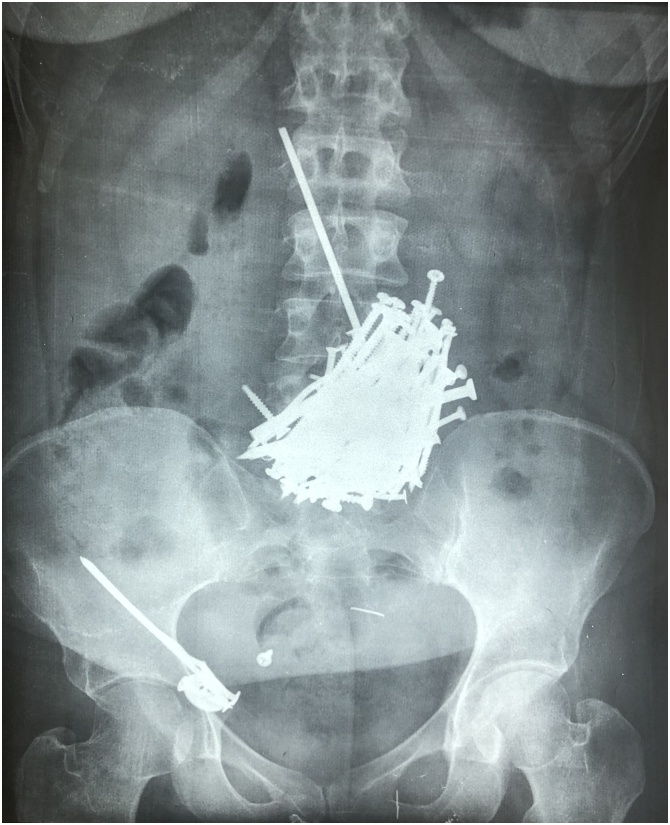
Plain abdominal X-rays of the abdomen showing multiple metallic nails in the stomach and the terminal ileum.
